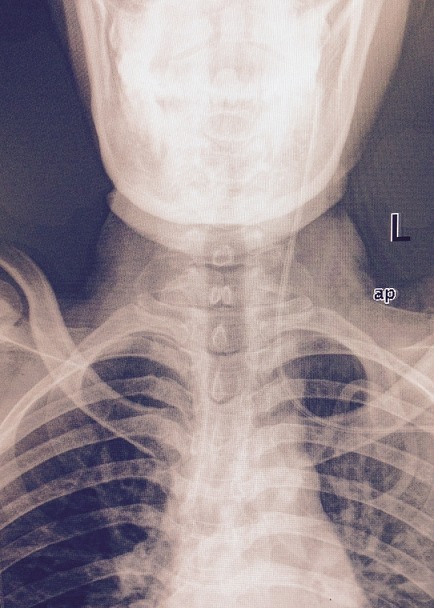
Patients Neck and Head X-ray
